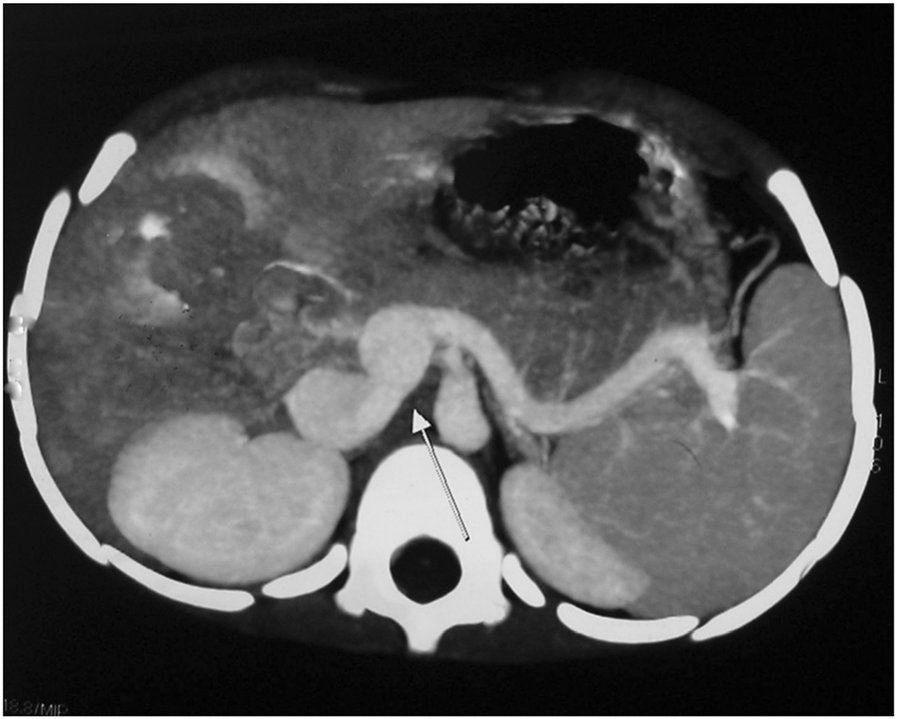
Contrast enhanced CT scan of upper abdomen with MIP to include of whole thickness of portal vein shows aberrant opening of portal vein into inferior vena cava (marked by white solid arrow in the figure). Note dilated inferior vena cava cranial to confluence in the image.
